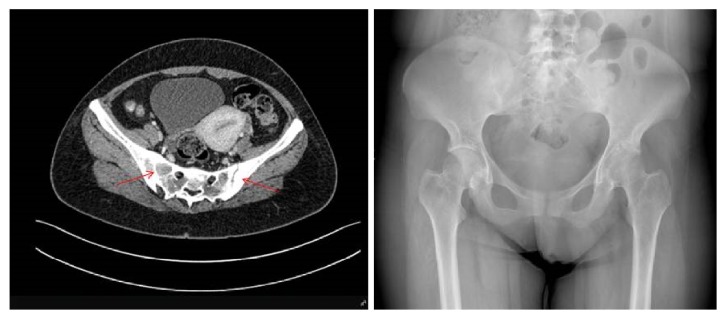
MRI and X-ray of sacroiliac joints showing total ankylosis of the right sacroiliac joint and partial ankylosis with irregularity on the nonsclerotic segments of the left sacroiliac joint (grade 3-4 sacroiliitis).
